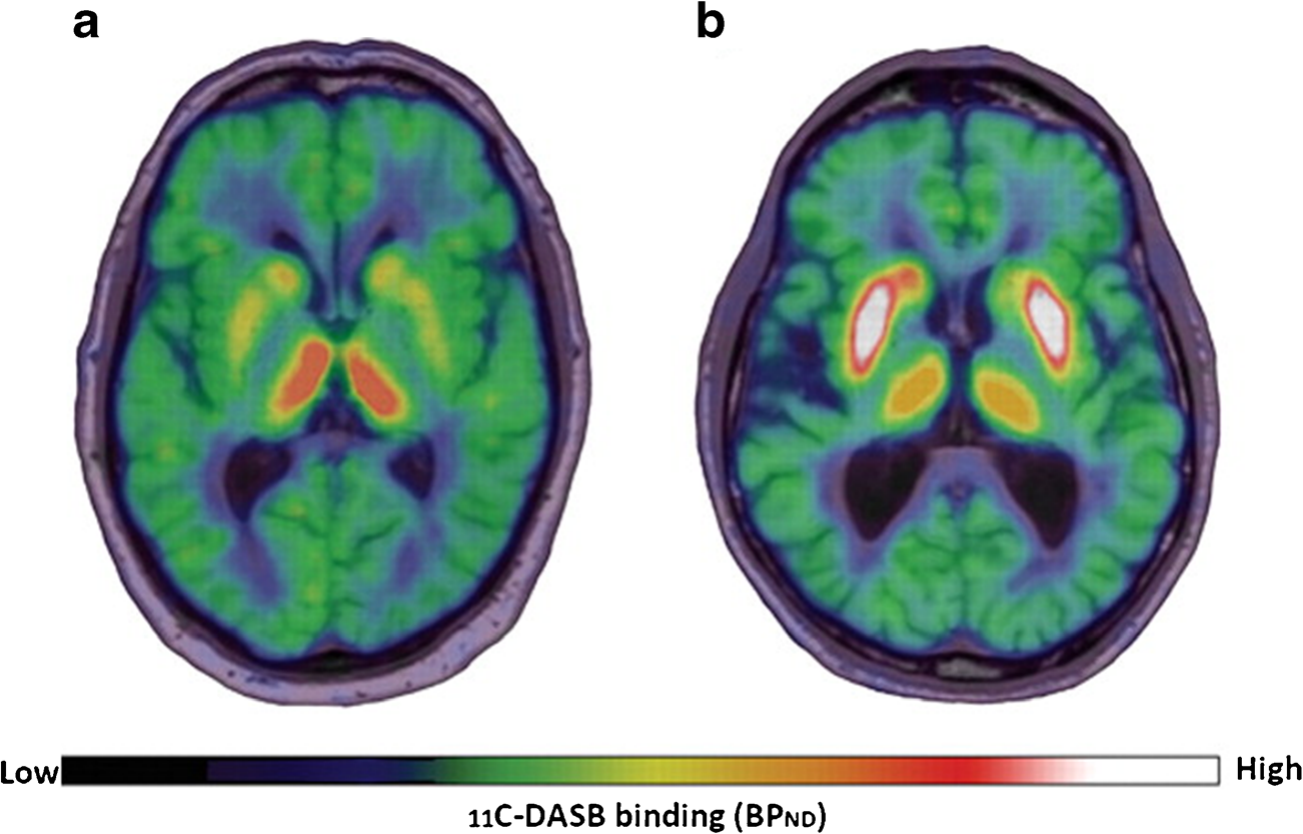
Role of striatal serotonergic hyperinnervation in graft-induced dyskinesia in Parkinson’s disease. ^11^C-DASB PET summation images of two Parkinson’s disease (PD) patients, co-registered on their individual MRI brain, showing their serotonin transporter (SERT) binding. a A 65-year-old male PD patient with 16 years disease duration and motor fluctuations, exhibiting reduced striatal ^11^C-DASB binding. b A 65-year-old male PD patient who received bilateral foetal striatal transplantation 16 years ago, experiencing graft-induced dyskinesia despite not taking any dopaminergic medications. His ^11^C-DASB PET showed significantly increased SERT binding in the striatum. Modified from Politis et al. [39]
